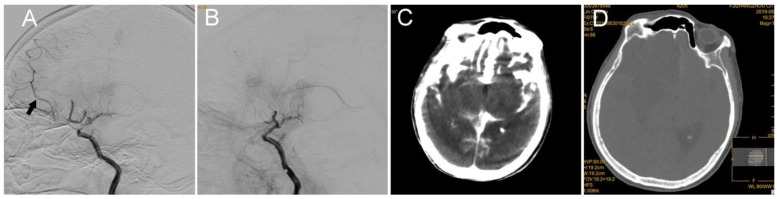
(A) Vessel perforation occurred when attempting to advance the microcatheter into A3; the microcatheter was in the parenchyma (the black arrow indicates the tip of the microcatheter). DSA shows the occlusion of the A2 segment of the left anterior cerebral artery. (B) This ruptured vessel was immediately sealed with 2 mL of thrombin (total dose was 300 u). (C) C-arm CT showed hyperdensity in the cisterna ambiens and sylvian cisterna. (D) Post-procedure dual-energy CT showed bilateral frontal lobe infarction without new hemorrhage or brain edema.
